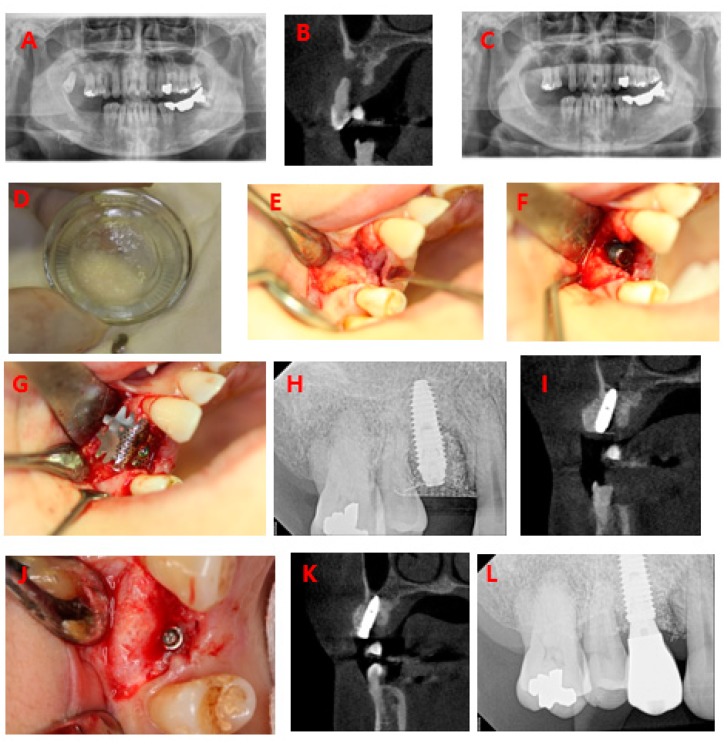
GBR case using AutoBT BMP. (A) Initial panoramic radiograph; (B) Initial CT view: severe alveolar bone resorption in the region of 14; (C) Panoramic radiograph after tooth extraction; (D) AutoBT; (E–G): First surgery in the region of 14; (E): Mucoperiosteal flap elevation; (F): Implant insertion and bone graft; (G) Titanium mesh covering; (H): Periapical radiograph after implantation; (I) CT view after implantation; (J) Second surgery; (K) CT view after second surgery; (L) Periapical radiograph after prosthetic loading.
